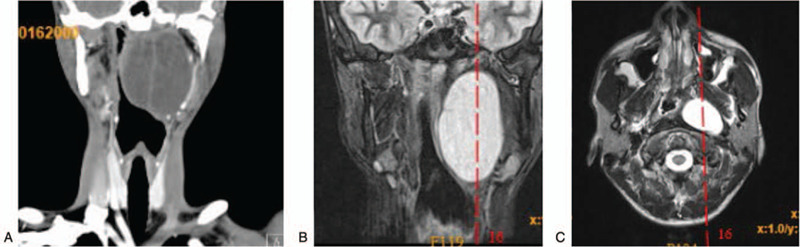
(A) Coronal computed tomography (CT) scan at first hospitalization showing a cystic lesion of 6.2 cm × 4.0 cm × 7.7 cm in the left parapharyngeal space, above the skull base, below the medial submandibular gland, protruding into the pharyngeal cavity, and resulting in pharyngeal cavity stenosis. (B–C) MRI (6 months later; second hospitalization) indicated that the dimensions of the left parapharyngeal space (left rear of the nasopharyngeal oropharynx plane) were approximately 5.1 cm × 2.9 cm × 7.6 cm with abnormal signal shadow, which is consistent with cystic lesions, protruding into the pharyngeal cavity, and resulting in pharyngeal cavity stenosis.
